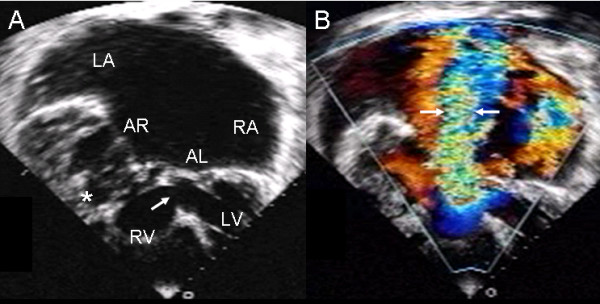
Atrioventricular septal defect with Rastelli type C common valve in situs inversus. (A) Two-dimensional 4 chamber image shows situs inversus and the insertion of the anterior leaflets to two papillary muscles (asterisk) joined to the right ventricular free wall. The arrow points to the ventricular septal defect beneath the leaflet bridge. (B) With color Doppler the severe regurgitation of the common atrioventricular valve can be seen (arrows) Abbreviations as before.
